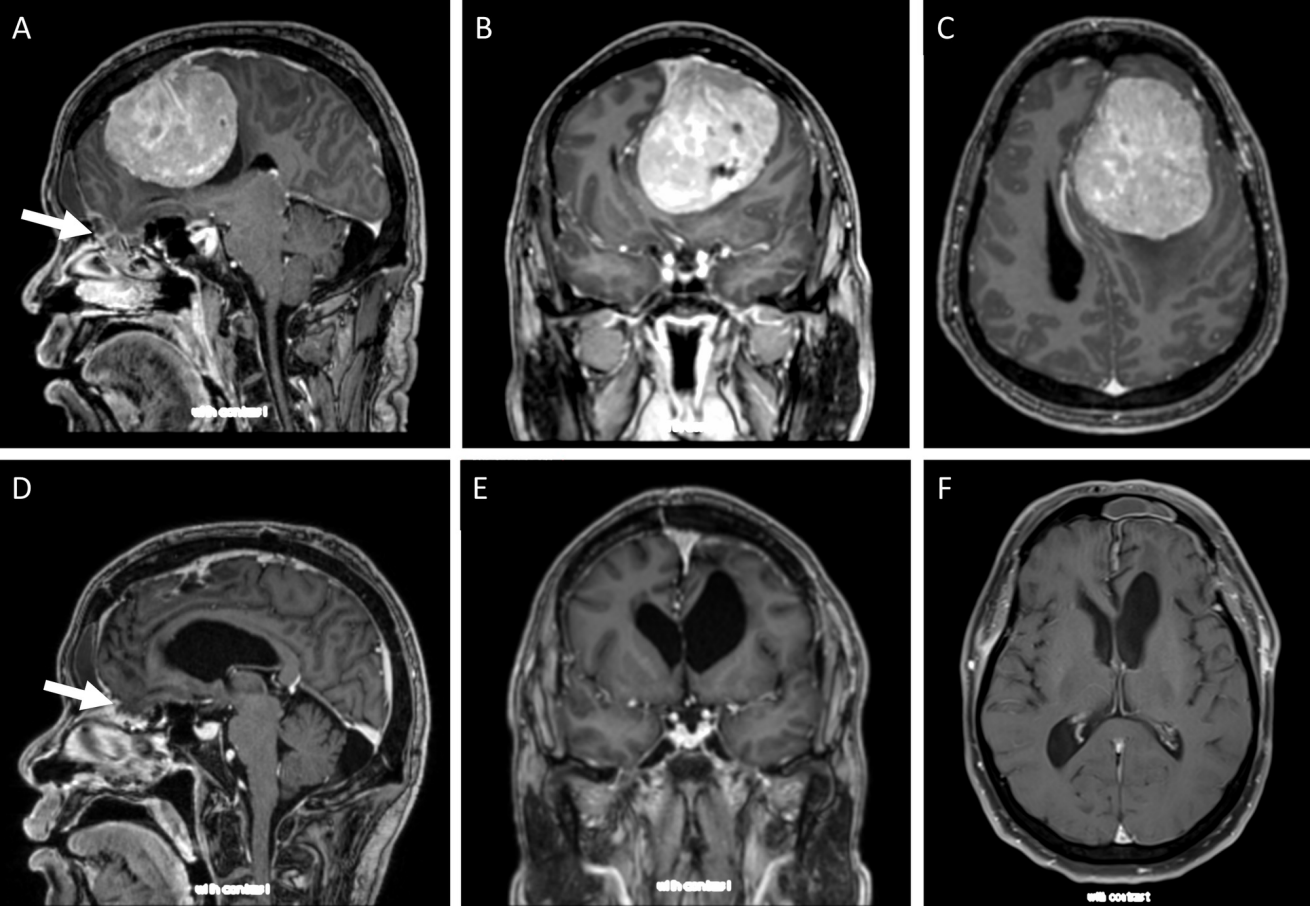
(A-C) Preoperative and (D-F) postoperative MRI of giant meningioma. Note small encephalocele (white arrows), remote from resection bed. MRI, magnetic resonance imaging
